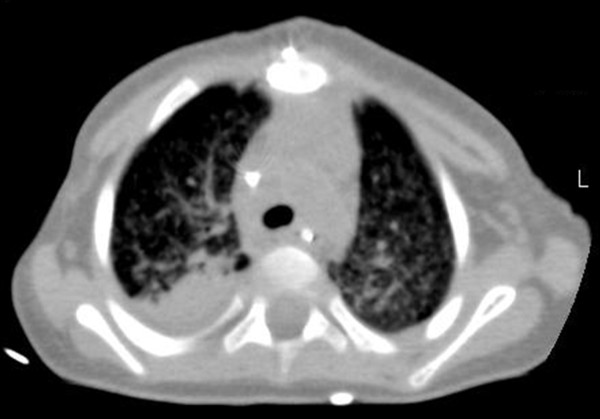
Chest Computed Tomography. Innumerable tiny, well-defined, miliary nodules throughout the lungs, and bronchovascular structures, a consolidation with air bronchogram in the posterior segment of the right upper lobe and an initial consolidation in the lateral segment of the right inferior lobe with evident local bronchiectasis.
